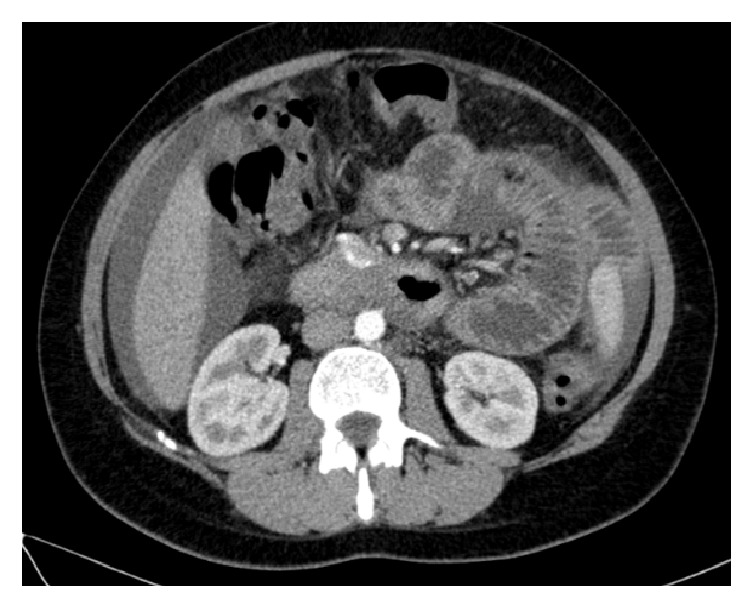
CT scan showing ascites and thickening and distension of small bowel loops.
